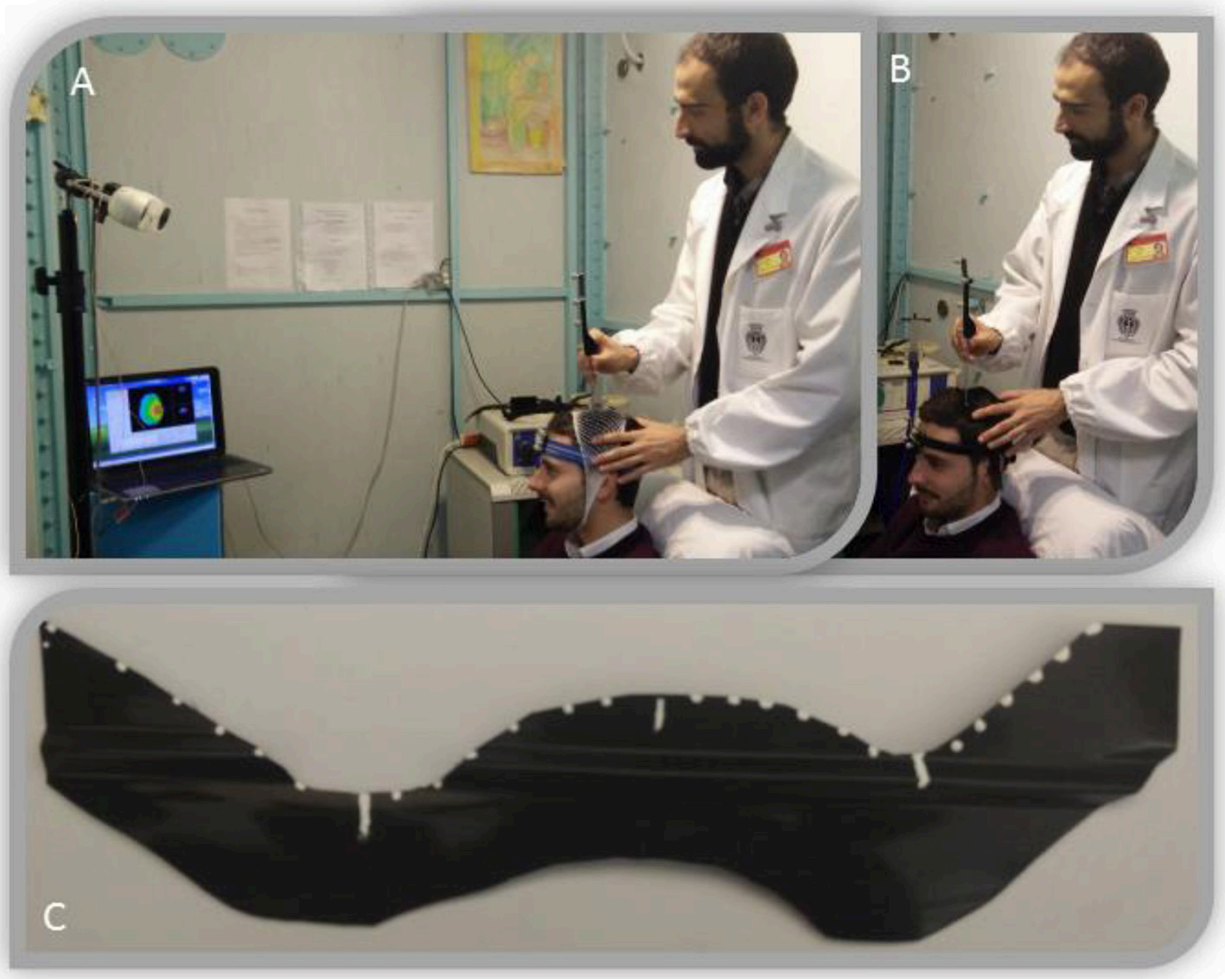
Neuronavigation setup used to estimate RePE positioning systems. Top: As detailed in the Methods, experimental neuronavigated procedure to test the RePE positioning via the MRI-derived 3D-head model measures (A) and via the AHF (B). Informed consent was obtained from the participants executing the procedure and wearing RePE to publish the image. (C) RePE with the testing point used for the neuronavigated procedure.
